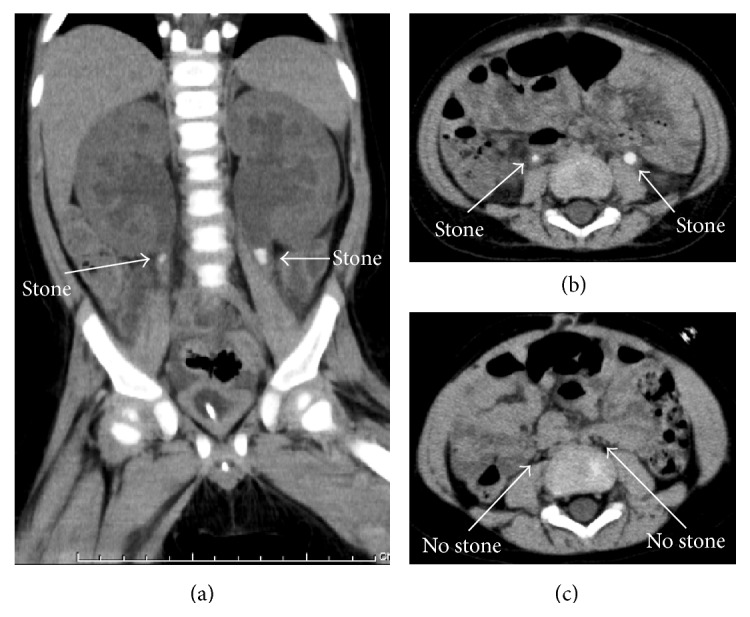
Computed tomography (CT) images for Case 3 are shown. (a) A coronal CT section revealed ureteral stones with hydronephrosis in the bilateral ureters (arrows). (b) A transverse CT section revealed ureteral stones with hydronephrosis in the bilateral ureters (arrows). (c) A transverse CT section after treatment is shown. The ureteral stones vanished and the hydronephrosis was resolved (arrows).
